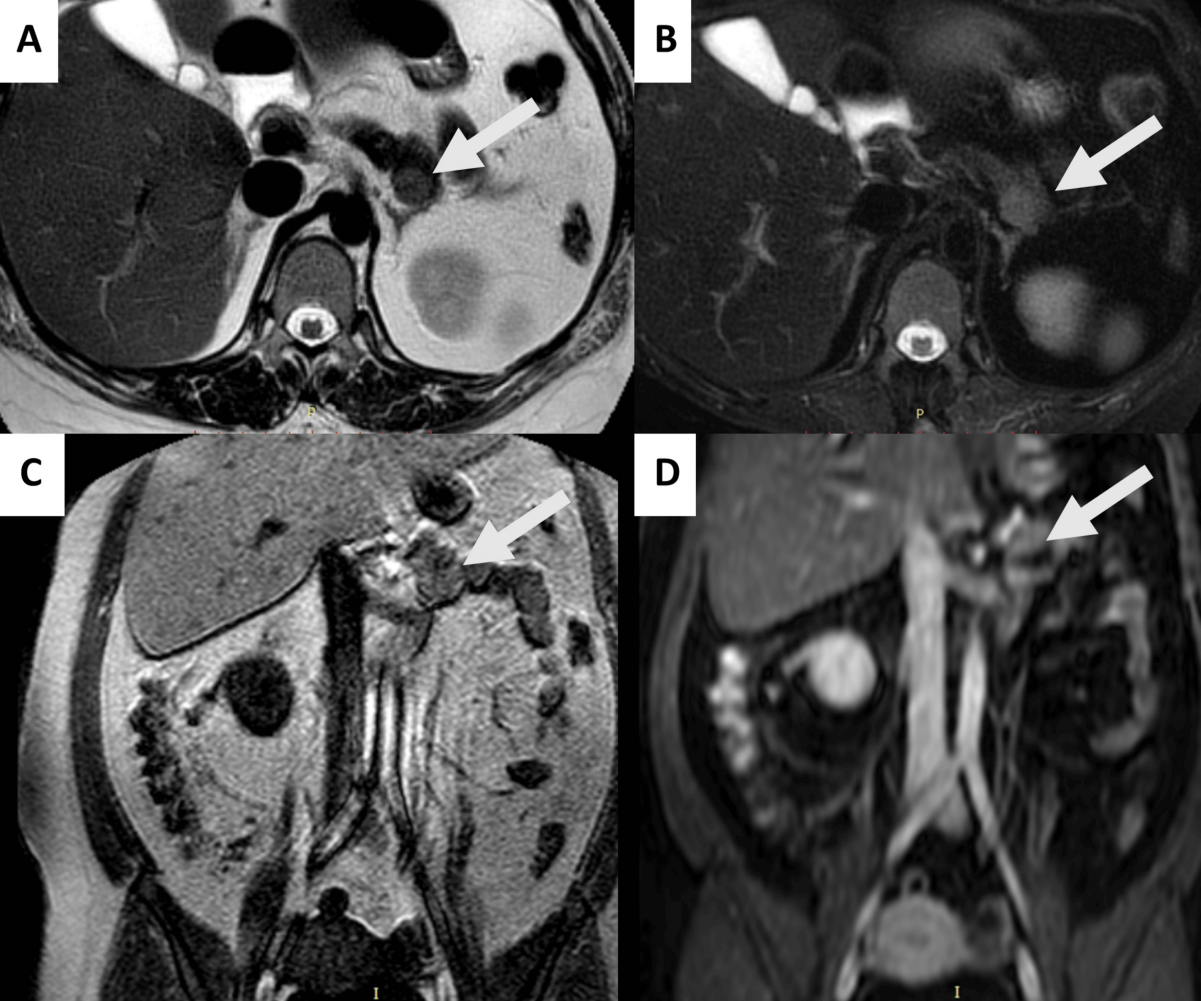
Abdominal MRI with intravenous contrast revealing a solid mass in the pancreatic tail (white arrow), which is round, well-defined, with regular margins, and measures 1.89 x 1.49 cm. The lesion appears slightly hyperintense compared to the surrounding normal pancreatic parenchyma on (A) axial T2-weighted image and (B) axial T2-weighted image with fat suppression. The lesion is isointense compared to the surrounding pancreatic parenchyma on (C) coronal T1-weighted image. No significant enhancement post-gadolinium contrast injection or infiltration into the adjacent structures is observed on (D) post-contrast coronal T1-weighted image.
